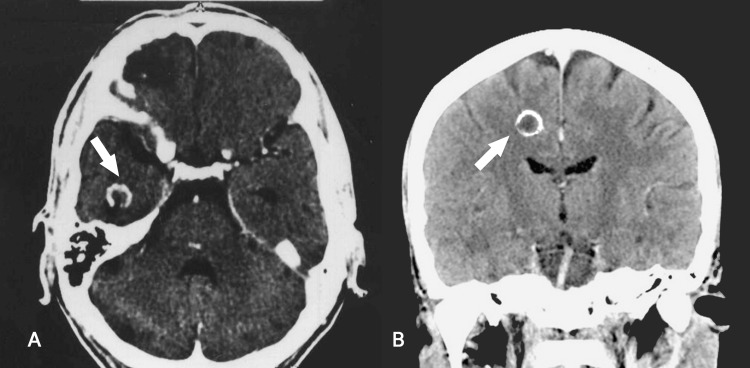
CT scan of the brain showing a ring-enhancing, intracerebral lesion (white arrow) in the (A) right temporal lobe and (B) parietal lobe.
